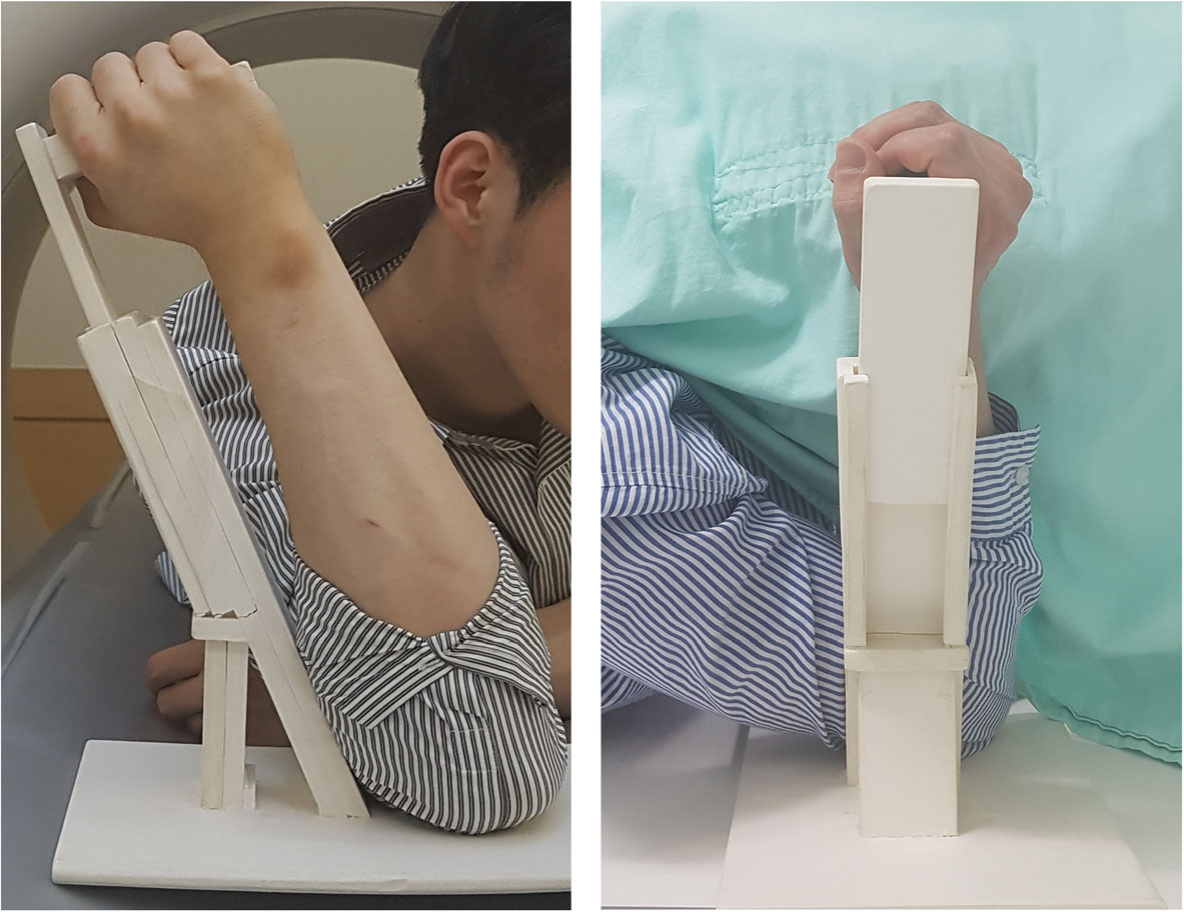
Patient with arm support system in CT machine. The center of the plate of the arm support system is located at the center of the patient’s bed. This arm support system can lengthened to ensure equal elbow flexion angle
